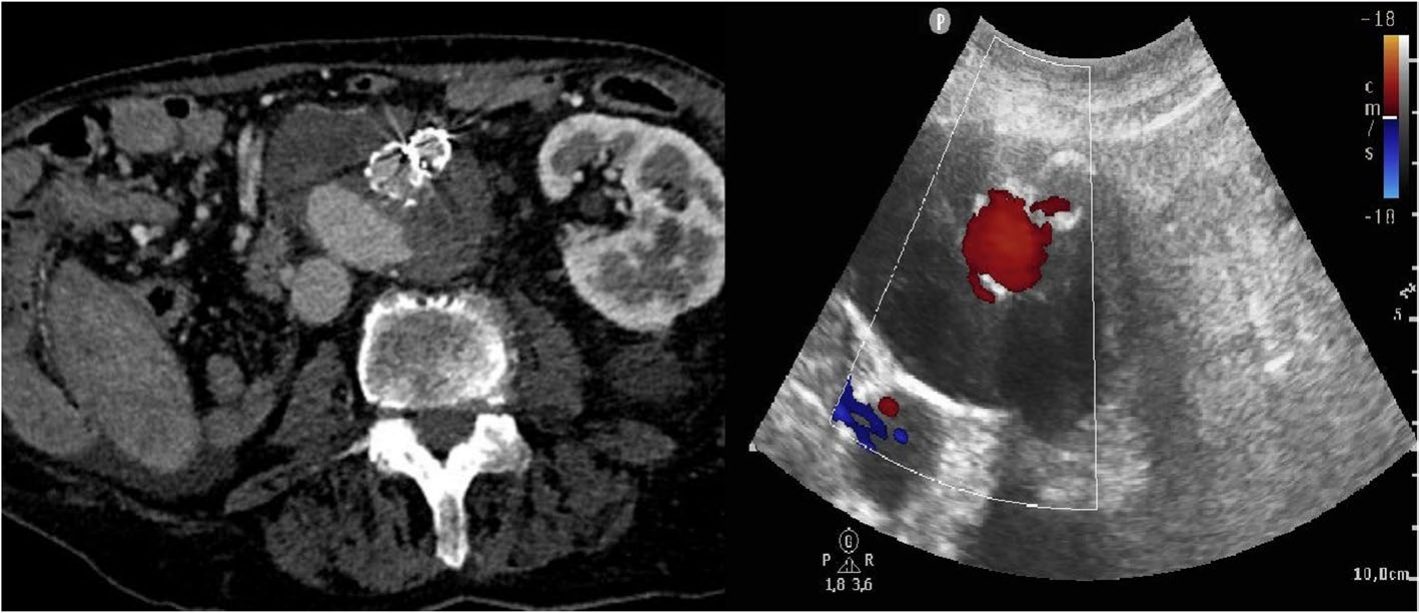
Type II endoleak demonstrated in the dorsal aspect of the aneurysm sac (CTA) (a). Transabdominal thrombin application was used to embolize type II endoleak (US) (b)
